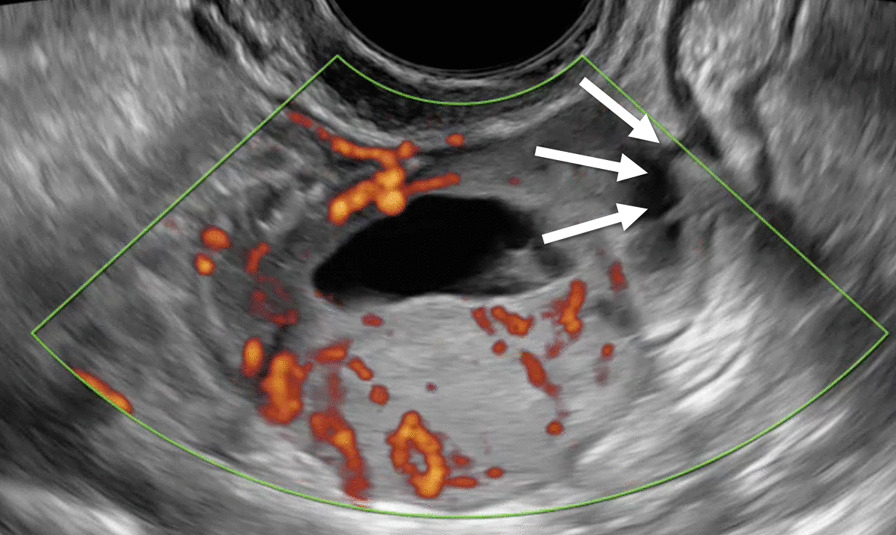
Power Doppler ultrasound image of the possible origin of the tumor
